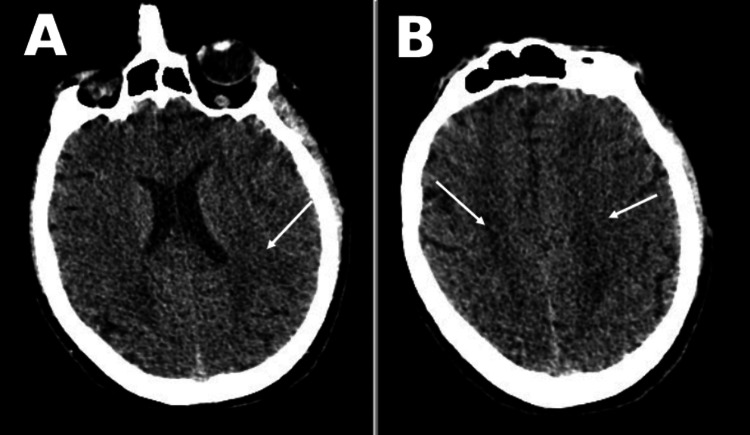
(A, B) Non-enhanced CT scan of the brain showing moderately decreased attenuation of the periventricular and the semiovale white matter (arrows).
